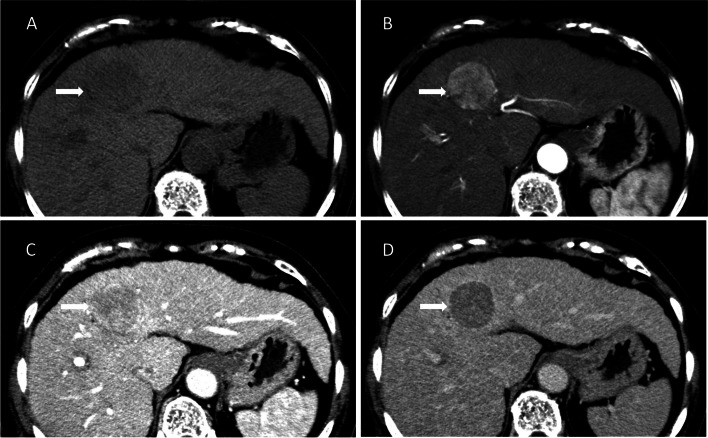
A Axial plain CT image shows a round hypoattenuating nodule in left hepatic lobe (arrow). B-D Axial contrast-enhanced CT in hepatic arterial phase (B) shows hyperenhancement and wash-out appearance in portal venous phase (C) and delayed phase (D)
